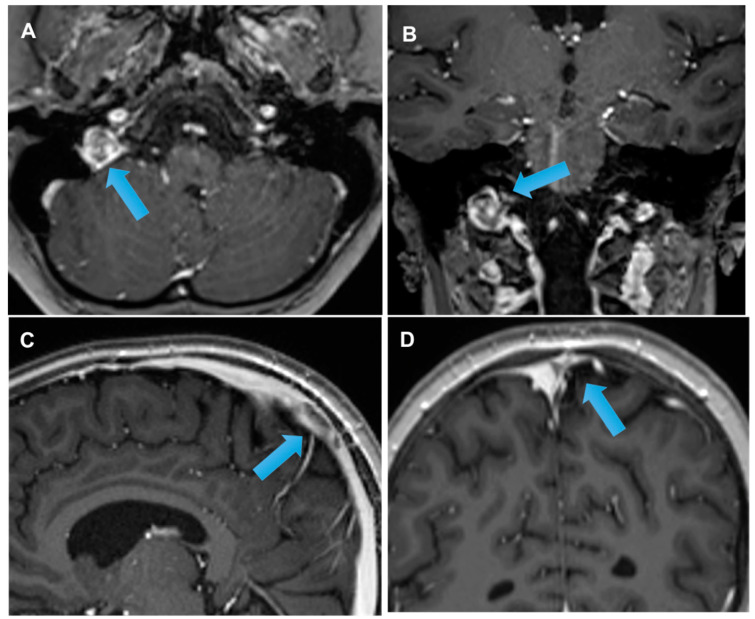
Patient affected by Hodgkin lymphoma during treatment. T1 w MRI after gadolinium administration demonstrates the presence of a complete venous thrombosis of the right jugular vein (arrow, A,B) and a non-complete thrombus into the superior sagittal sinus on the T1 sagittal view (arrow, C) and coronal view (arrow, D).
